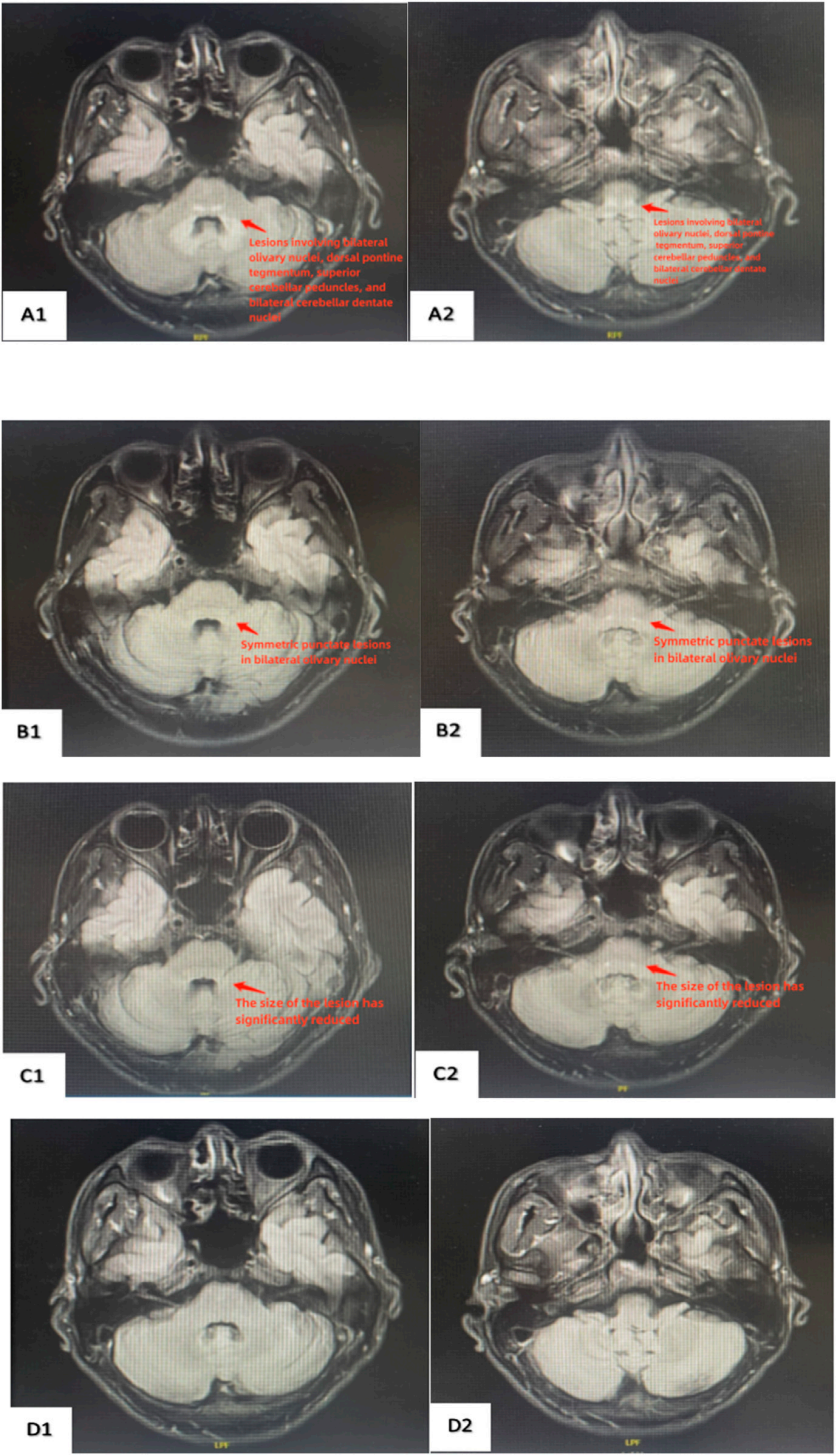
Dynamic changes in brain MRI before and after treatment. (A1,A2) (2023.11.4): Symmetrical abnormal signals in the bilateral olivary nuclei, tegmental pontine region, superior cerebellar peduncles, and bilateral dentate nuclei of the cerebellum. (B1,B2) (2023.11.13): Symmetrical abnormal signals in the bilateral olivary nuclei. (C1,C2) (2023.11.20): The abnormal symmetrical signals in the bilateral olivary nuclei have significantly decreased in size compared to previous scans, with some areas no longer clearly visible. (D1,D2) (2024.3.12): Abnormal symmetry signal of bilateral olivary nuclei have disappeared.
